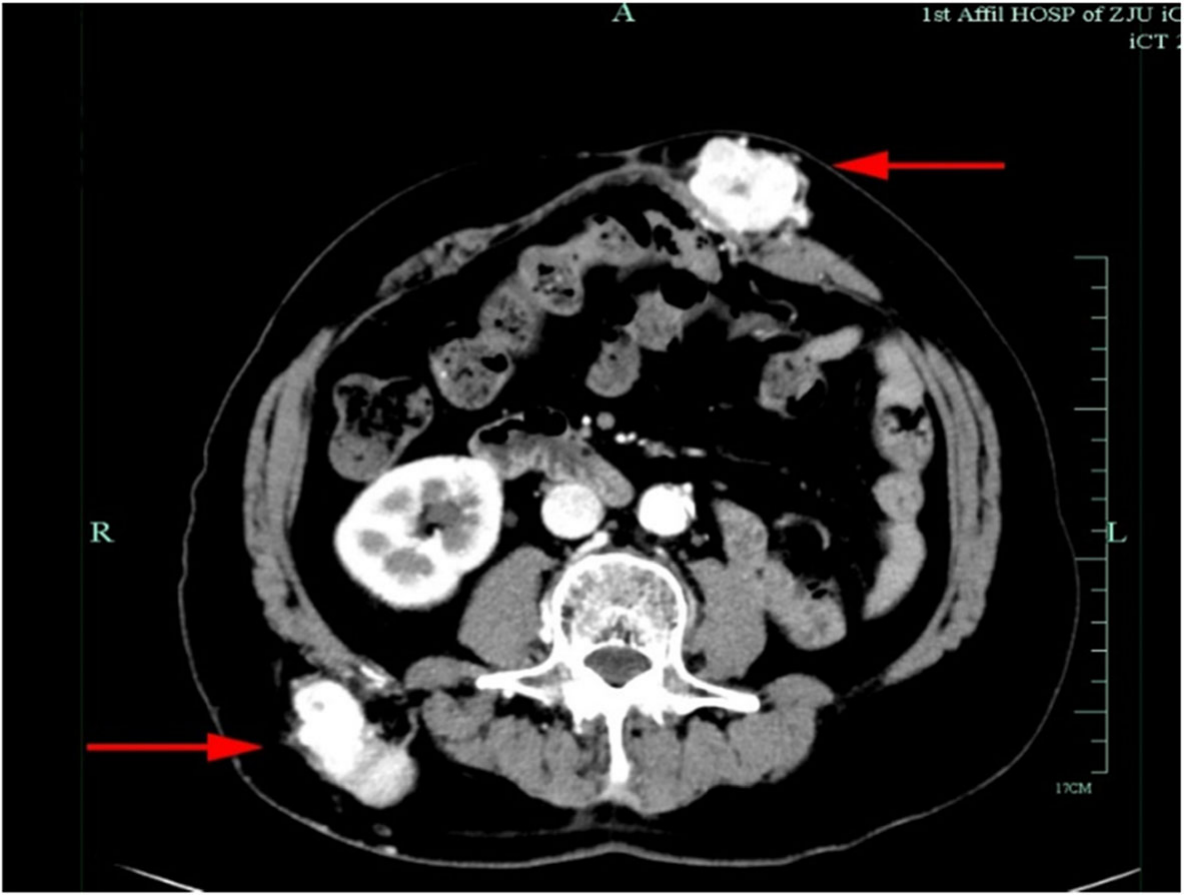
Enhanced computed tomographic scan showing a 5 × 6-cm mass in the left side of the anterior abdominal wall (*left arrow*) and a 5 × 6-cm mass in the back of the right waist (*right arrow*)
